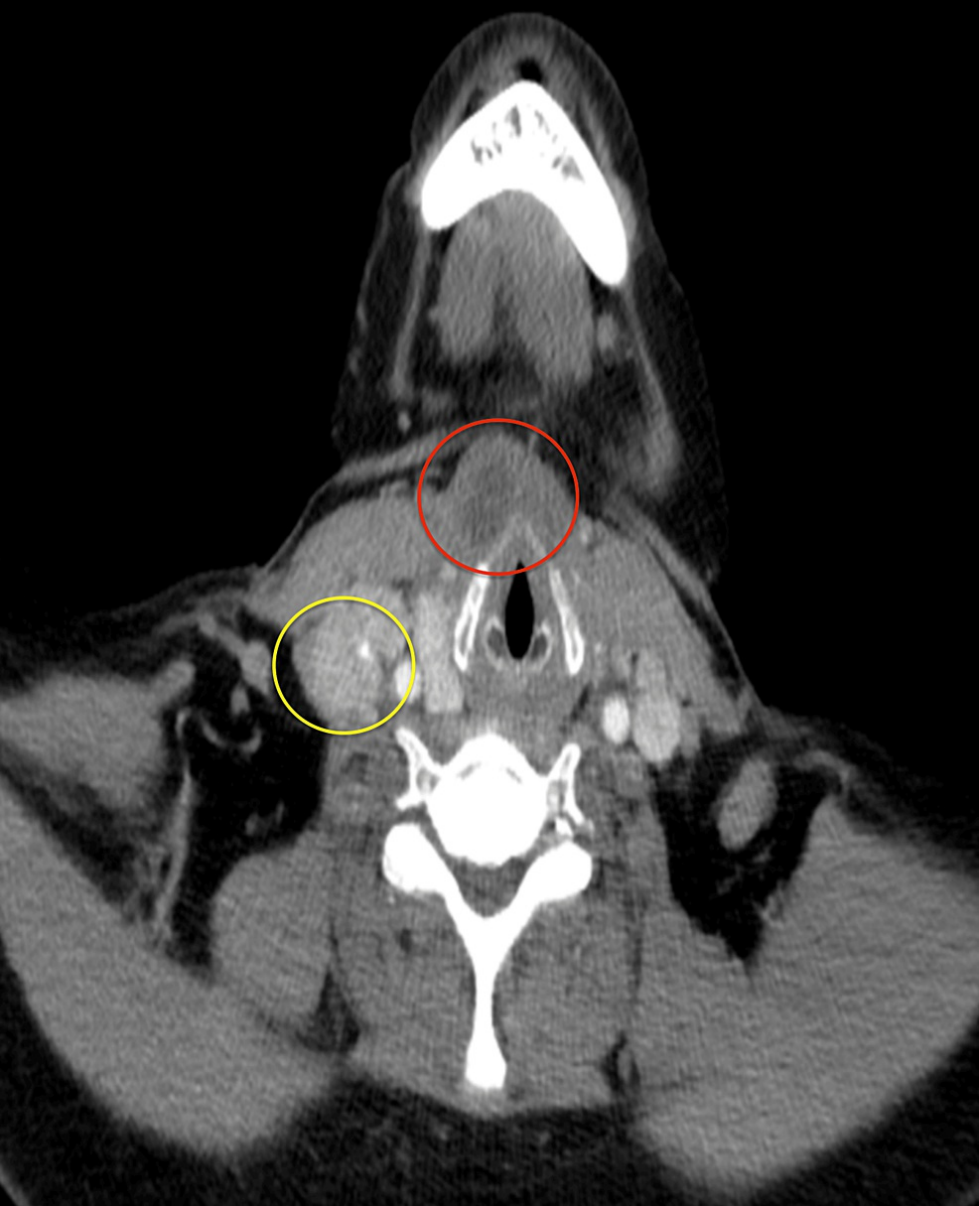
Axial-cut CT neck with contrast demonstrating both right lateral neck metastatic lymph node in levels III/IV and a prelaryngeal mass in the area of the pyramidal lobe for Patient 1 Yellow circle: metastatic lymphadenopathy in levels III/IV, red circle: thyroglossal duct cyst CT: computed tomography
